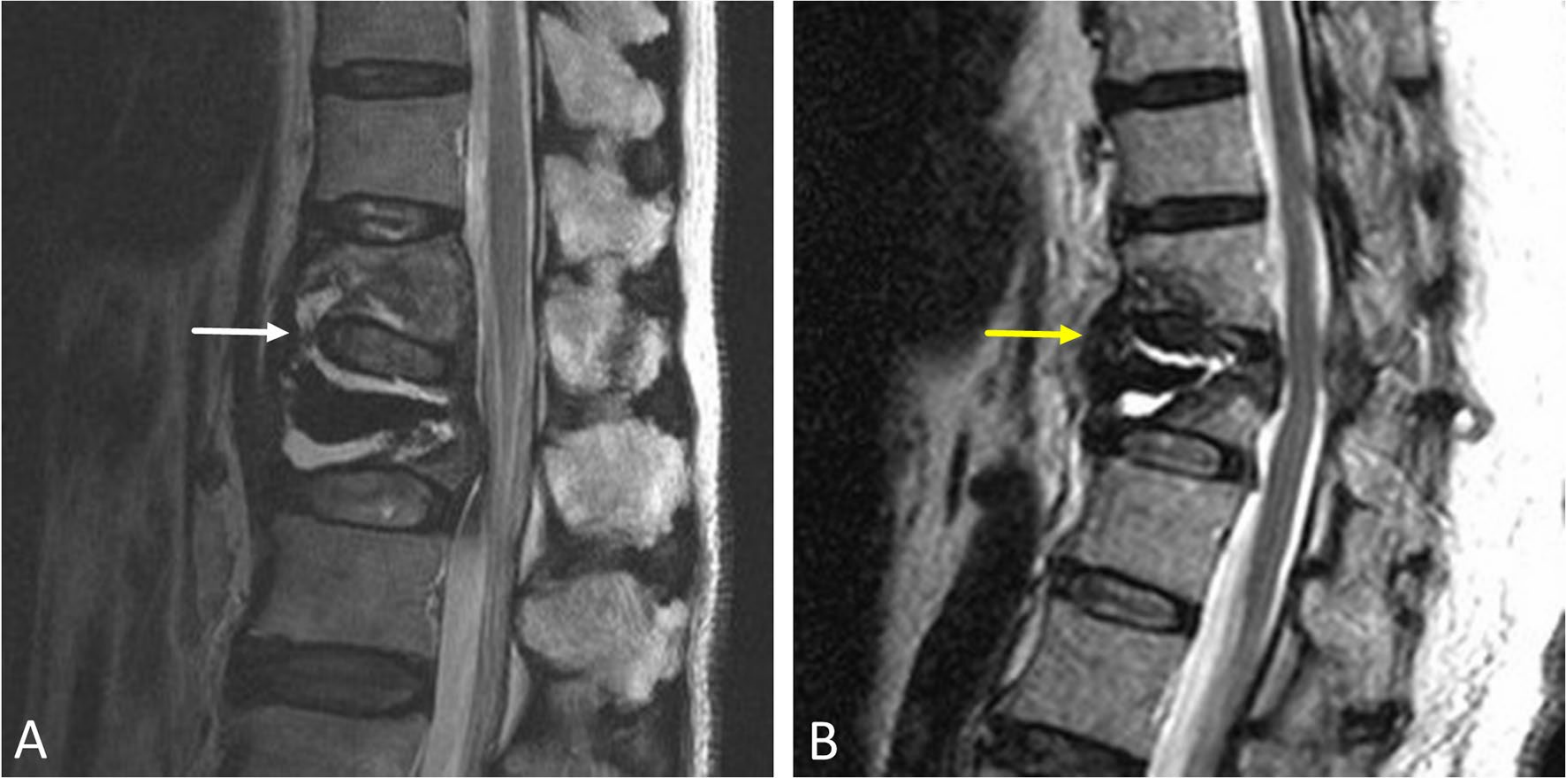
Preoperative MRI predictors of the cement bridging phenomenon include (A) fluid accumulation (white arrow) and (B) hypointense signaling (yellow arrow), indicating a redundant anterior longitudinal ligament, around the space anterior to the abutting vertebral bodies and the corresponding intervertebral disc.
